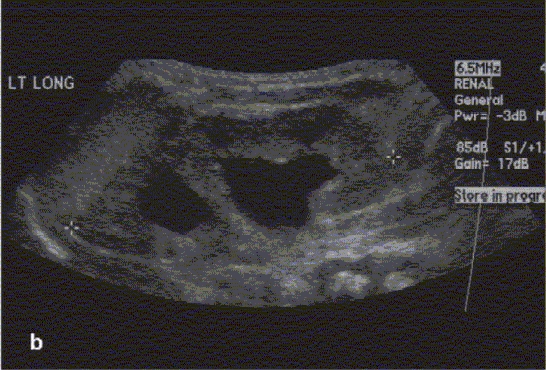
Renal ultrasound demonstrating left renal duplication
